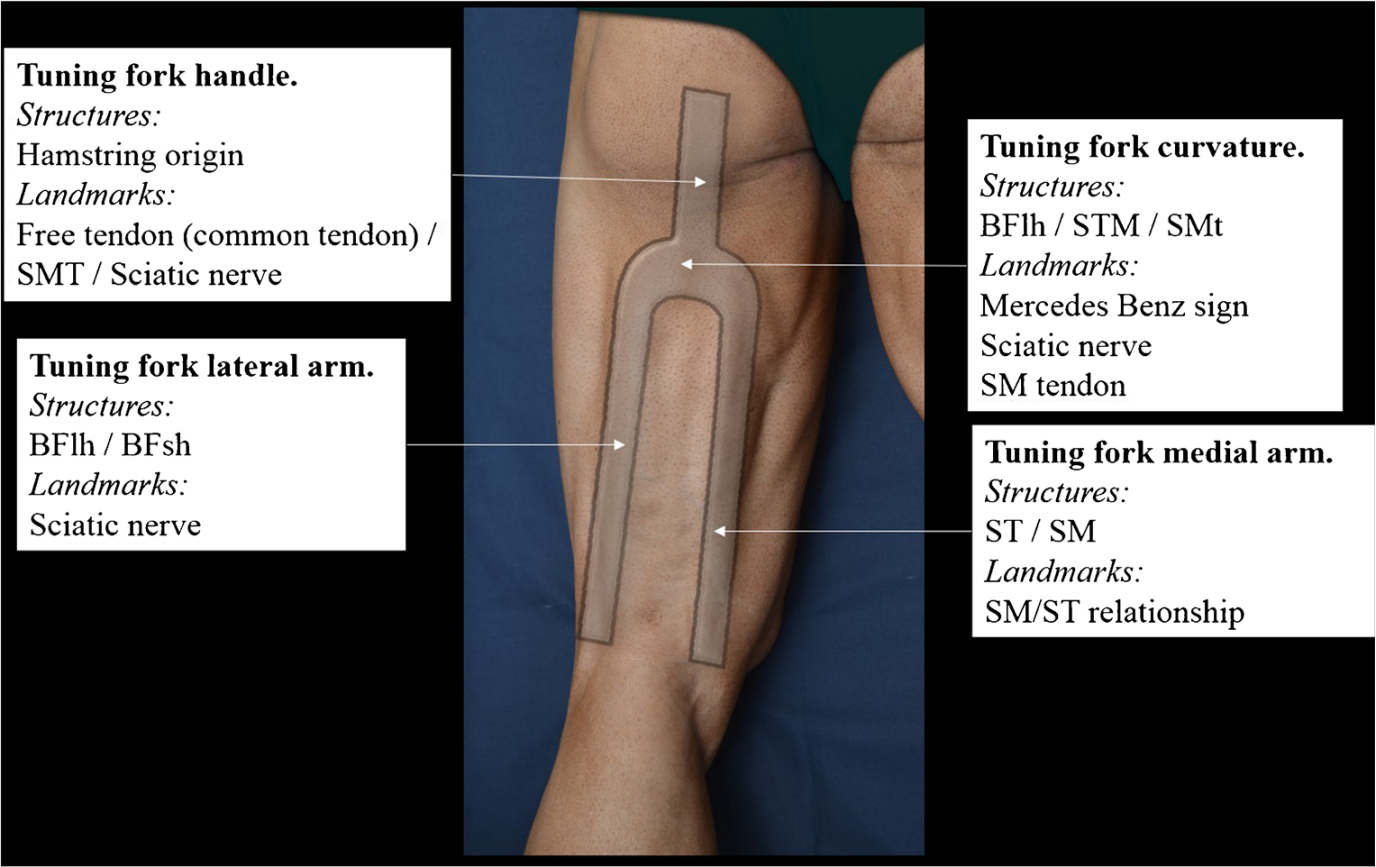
The hamstrings form an inverted tuning fork with four areas of study: the handle, the curve, and two arms. Each of these areas contains interesting anatomical structures and specific ultrasound landmarks that facilitate a systematic study of the hamstrings. *BFlh* long head of the biceps femoris muscle, *BFsh* short head of the biceps femoris muscle, *ST* semitendinosus muscle, *SM* semimembranosus muscle, *SMT* semimembranosus tendon
